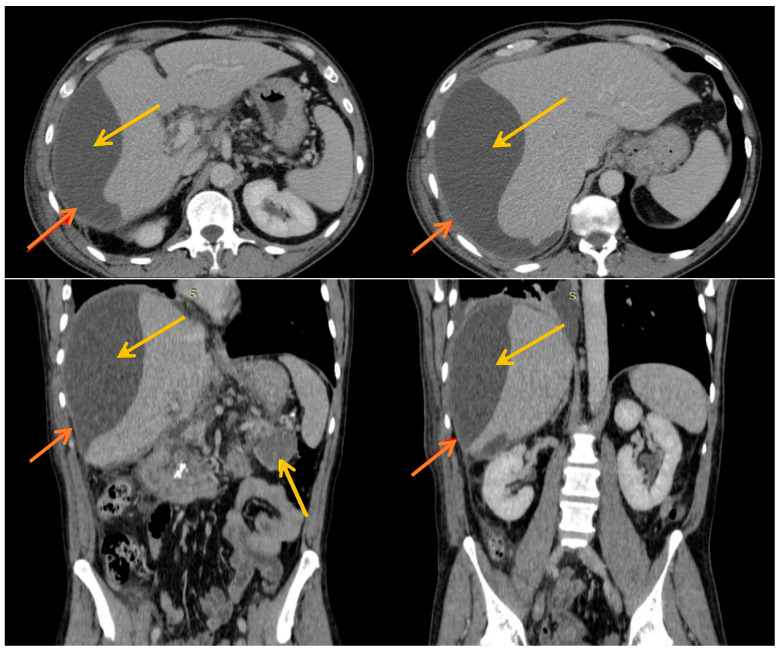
CT scan images: yellow arrow = pseudocyst; red arrow = hepatic capsule. The biconvex lens form specific for the hepatic subcapsular pseudocyst is observed.
